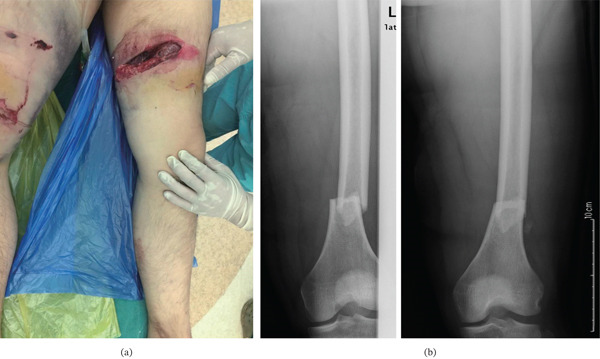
(a) A male patient suffered the open femoral shaft fracture due to a traffic accident. (b) Preoperative radiographs: oblique (left) and anteroposterior (right) views showing a transverse fracture of the left femoral shaft (AO/OTA 32A3). The oblique view was obtained due to patient positioning constraints.
